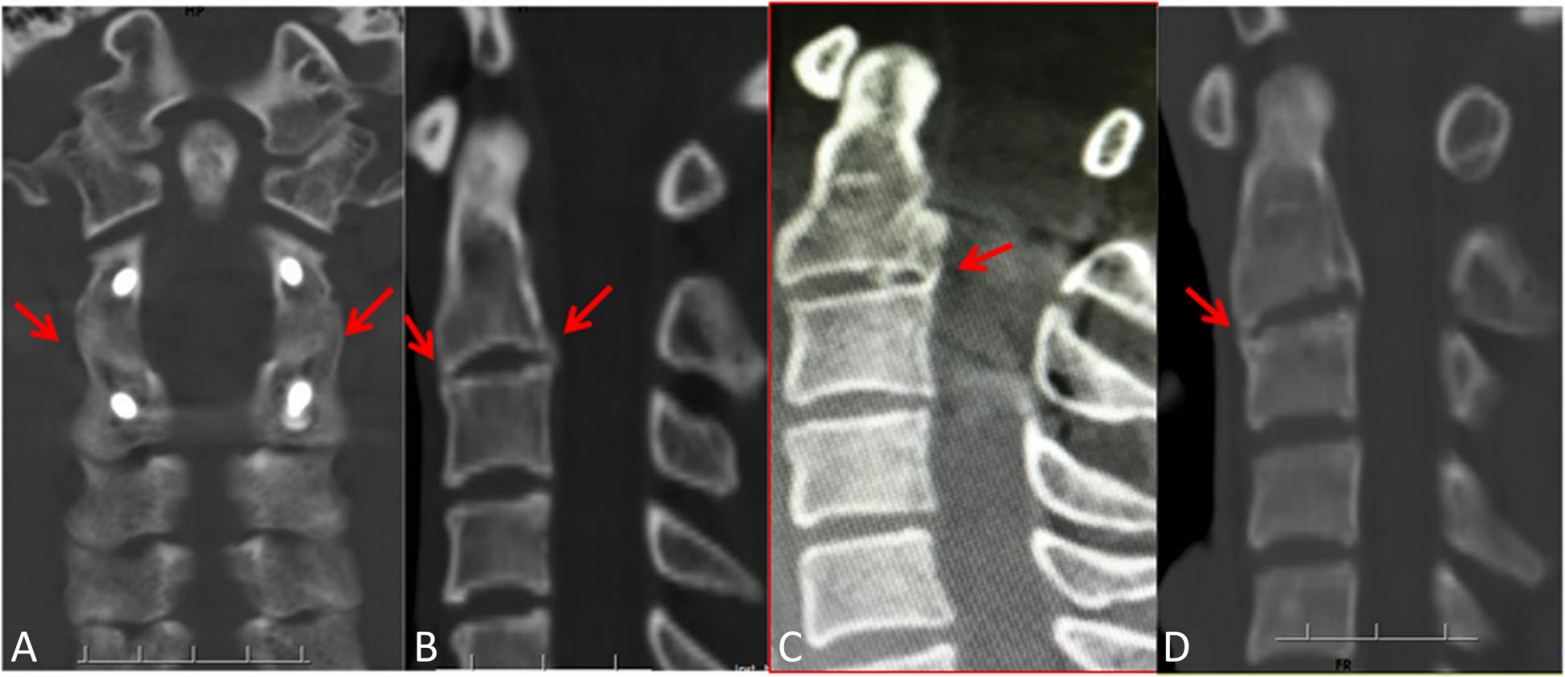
CT scans showing the spontaneous fusion of C2-C3: A spontaneous fusion of at bilateral C2-C3 facet joints shown by the red arrow; B both anterior and posterior bony bridge at C2-C3 of the intervertebral bodies shown by the red arrow; C posterior bony bridge at C2-C3 of the intervertebral bodies shown by the red arrow; D anterior bony bridge at C2-C3 of the intervertebral bodies shown by the red arrow
